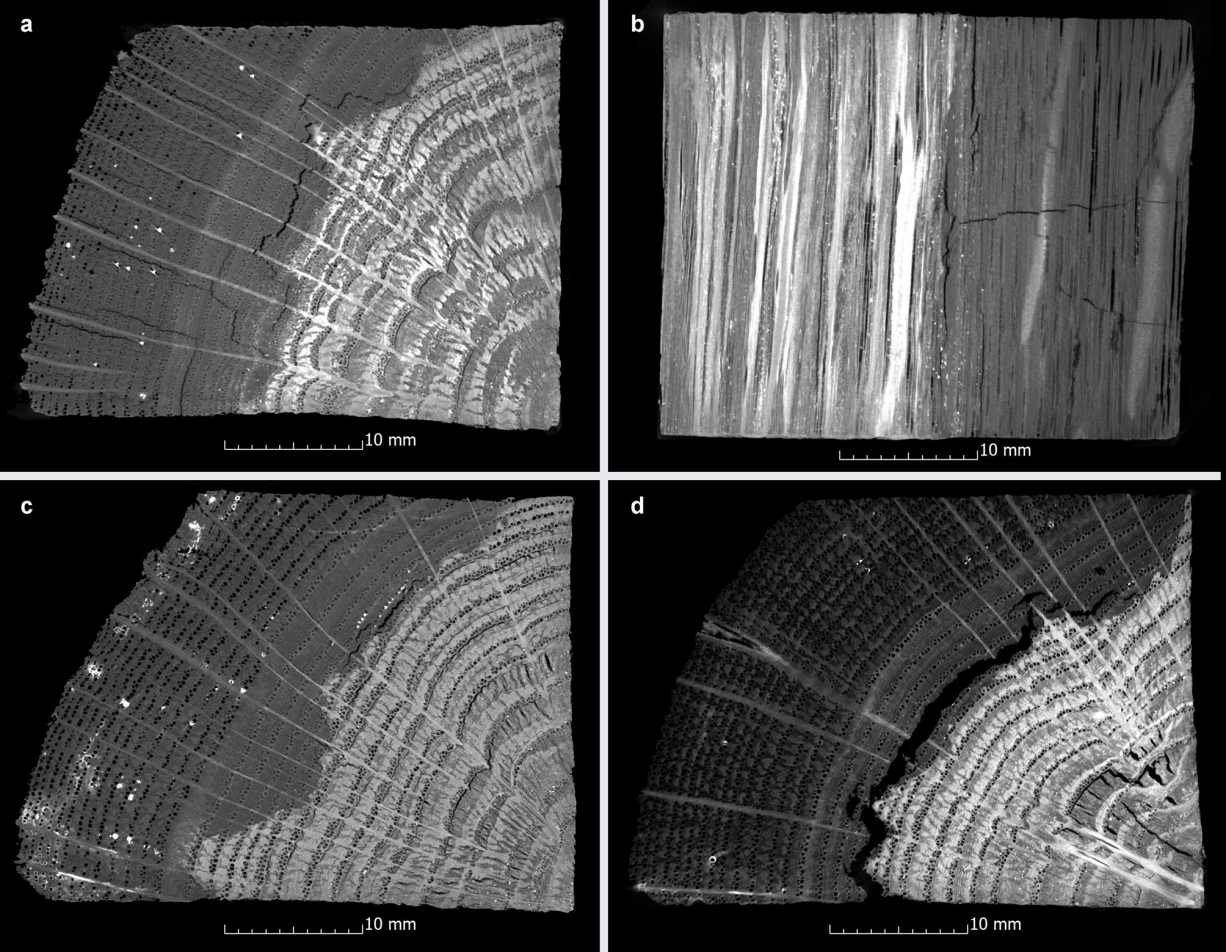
CT images of (a) radial, tangential and (b) transverse cracks in a PEG1 conserved oak sample (Oa-PEG1-4) as well as radial and tangential cracks in oak samples conserved with (c) PEG2 (Oa-PEG2-2) and (d) Kauramin 800 (Oa-K800-4).
